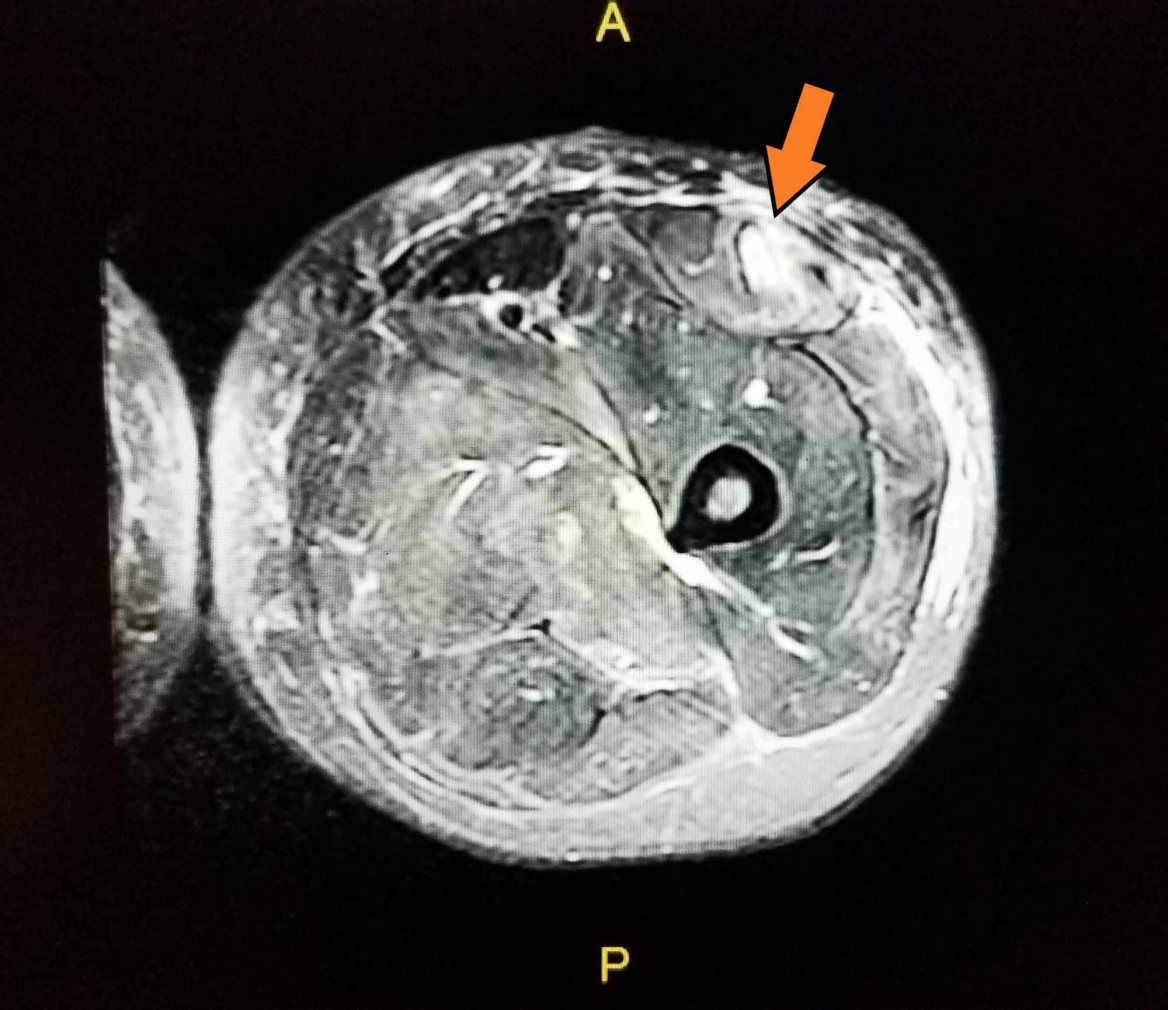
MRI of right thigh with an impaled piece of wood
